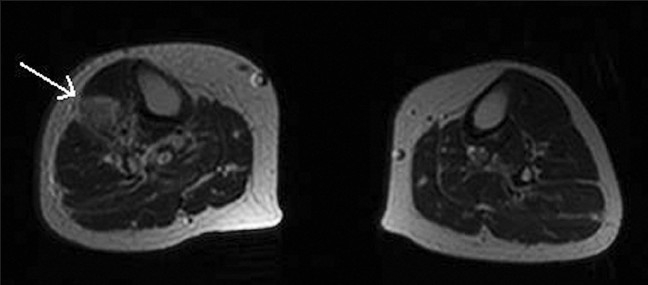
Axial MRI section of right leg showing extent of lesion infiltration down to the tibia
